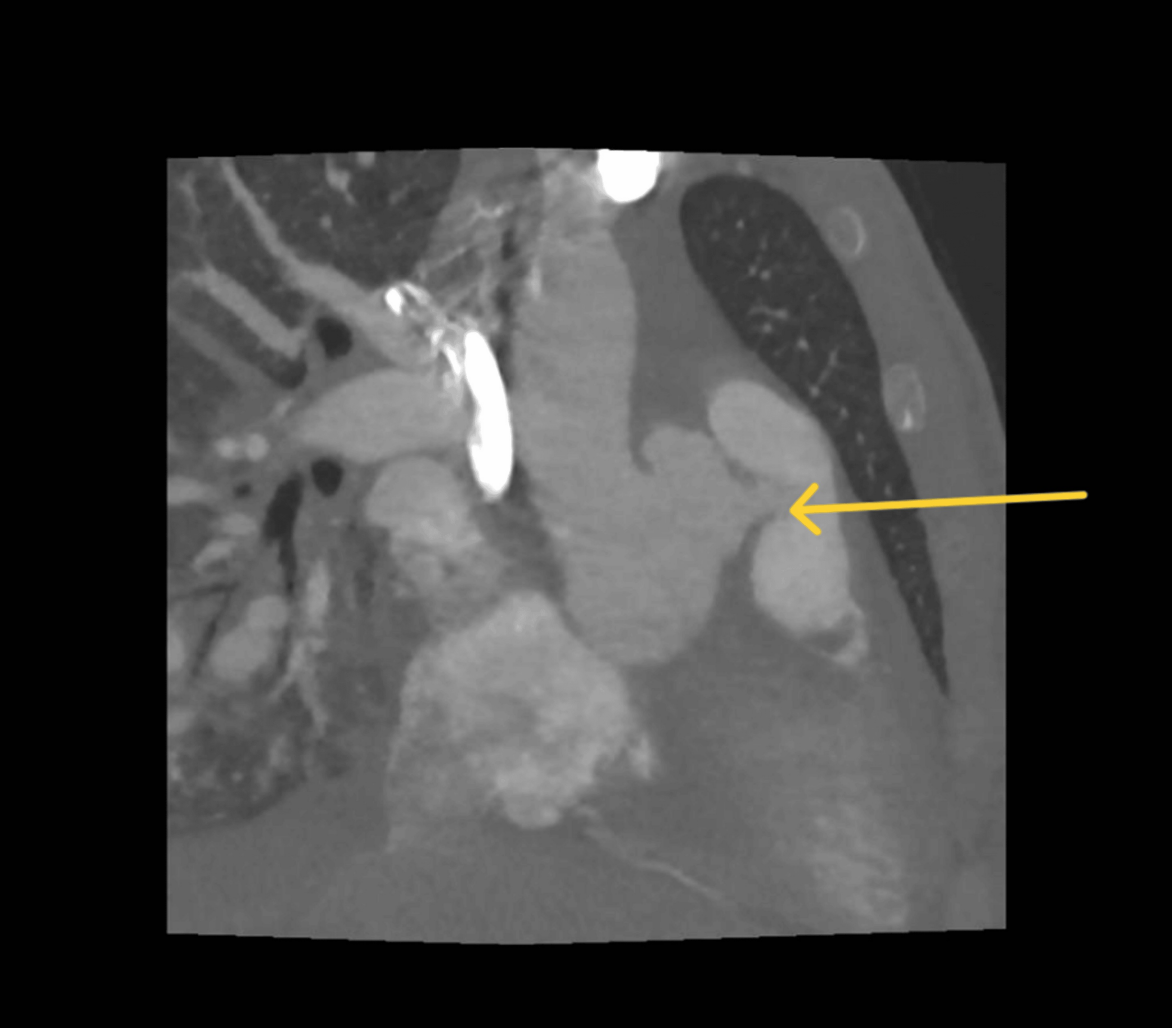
Sagittal CT scan of the lungs demonstrating a sinus of Valsalva aneurysm with a fistulous connection to the pulmonary trunk.
